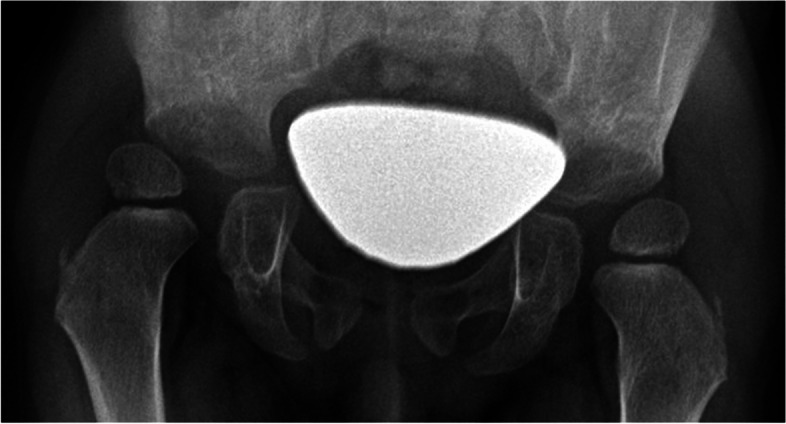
Anterioposterior hip radiograph for case 3 showing laterally displaced femoral capital epiphyses, poorly formed acetabula
